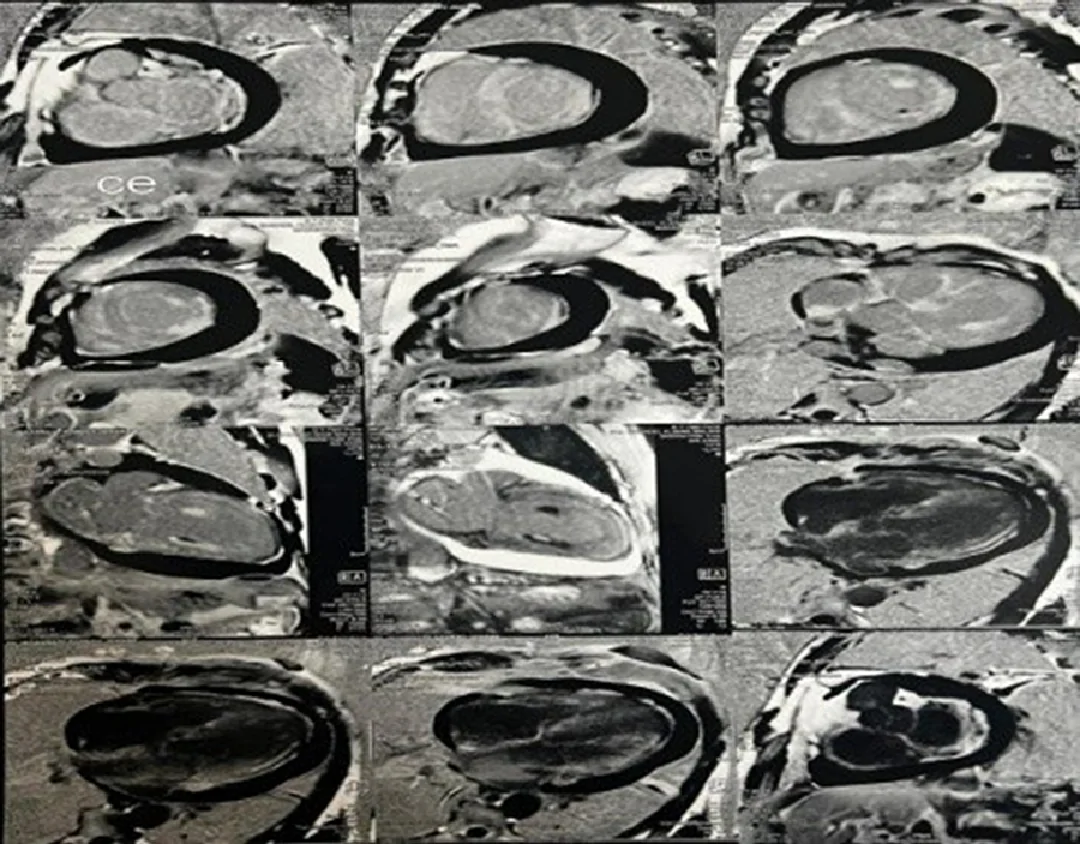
Cardiac magnetic resonance imaging (CMR) of transthyretin cardiac amyloidosis. Representative late gadolinium enhancement (LGE) images obtained in long-axis and short-axis planes demonstrate diffuse concentric left ventricular wall thickening, biatrial enlargement, and extensive circumferential subendocardial LGE, consistent with diffuse myocardial amyloid infiltration.
